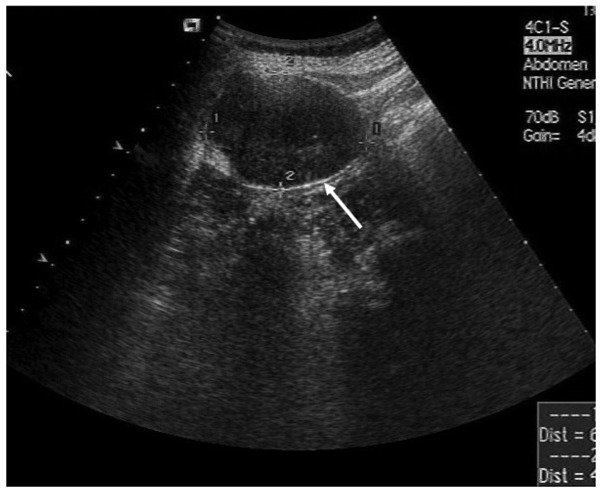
B-mode ultrasound showing the well-encapsulated mass in the anterior abdominal wall (arrow).
